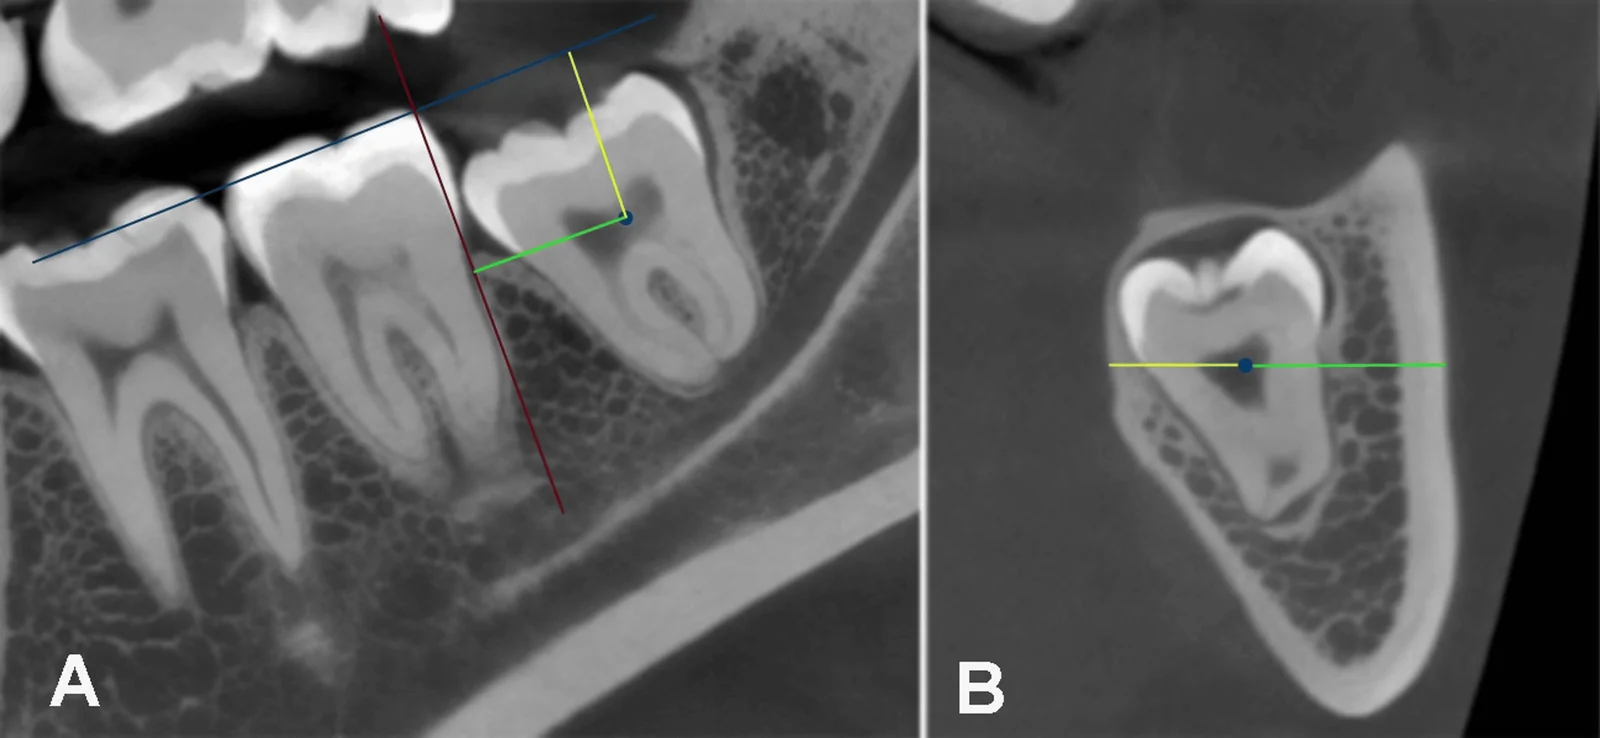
Cone-beam CT image of impacted mandibular third molar The image shows (A) anteroposterior length (green line) and vertical depth (yellow line) in sagittal view, (B) buccal (green line) and lingual (yellow line) width in axial view Blue line: occlusal plane; red line: distal surface of second mandibular molar; blue dot: central reference point on impacted mandibular third molar CT: computed tomography
